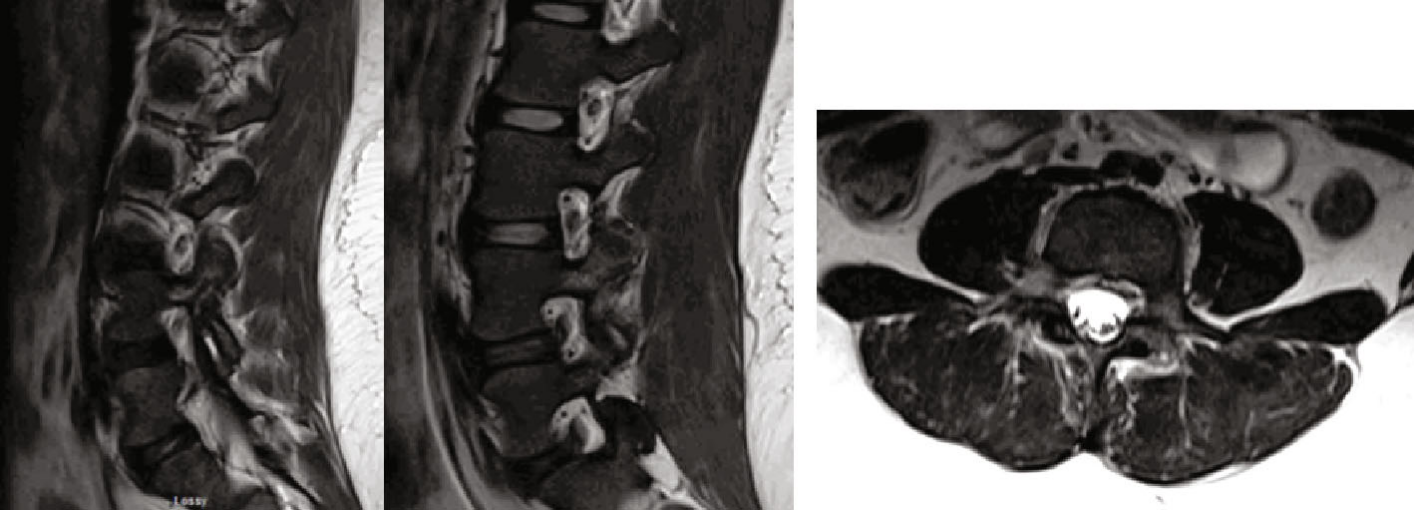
MRI without contrast, T2 sequence, sagittal and axial cut, with a bilateral L4 pars defect with associated bone marrow edema.
